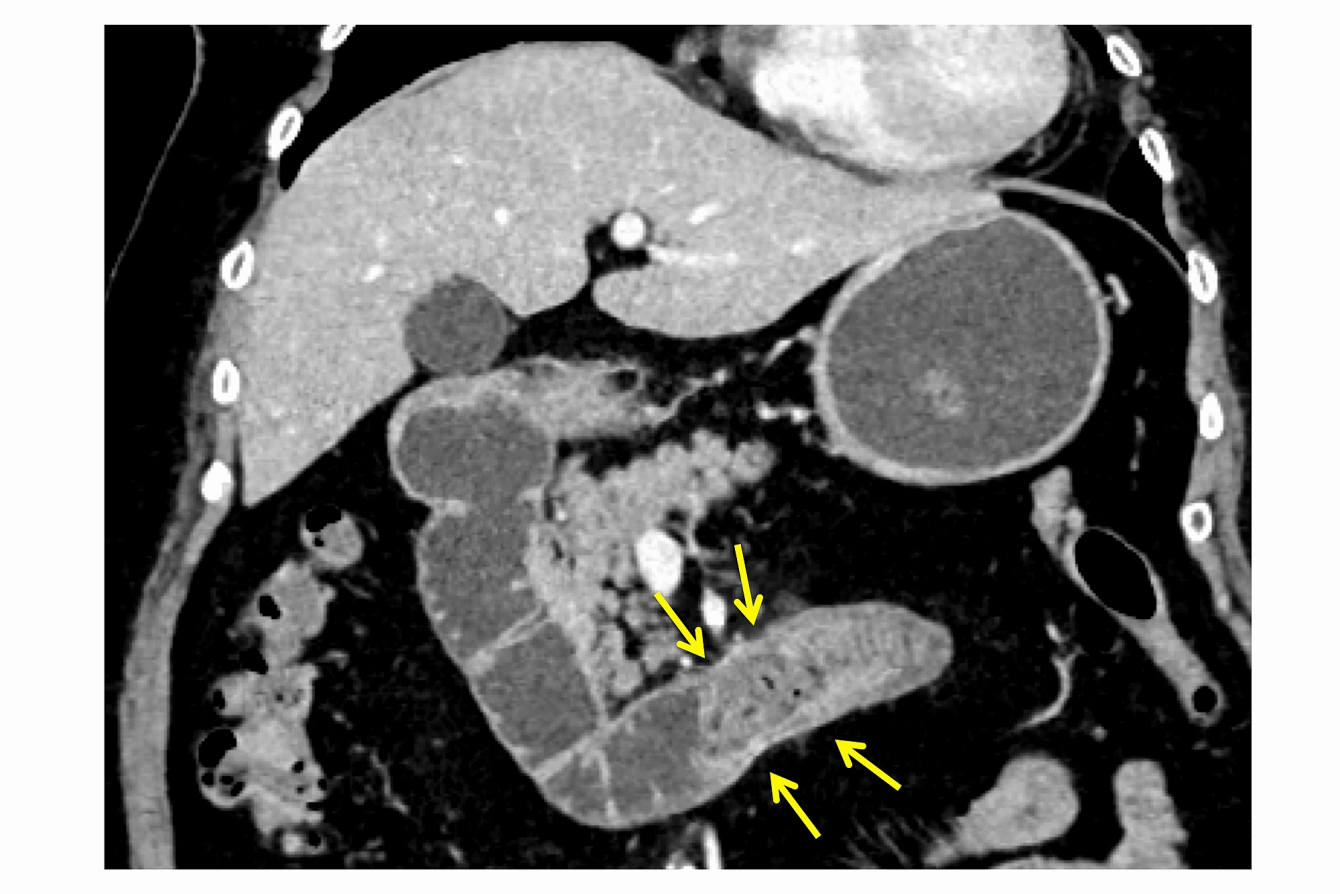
Abdominal computed tomography at admission Bowel obstruction is seen in the third portion of the duodenum (yellow arrows).
